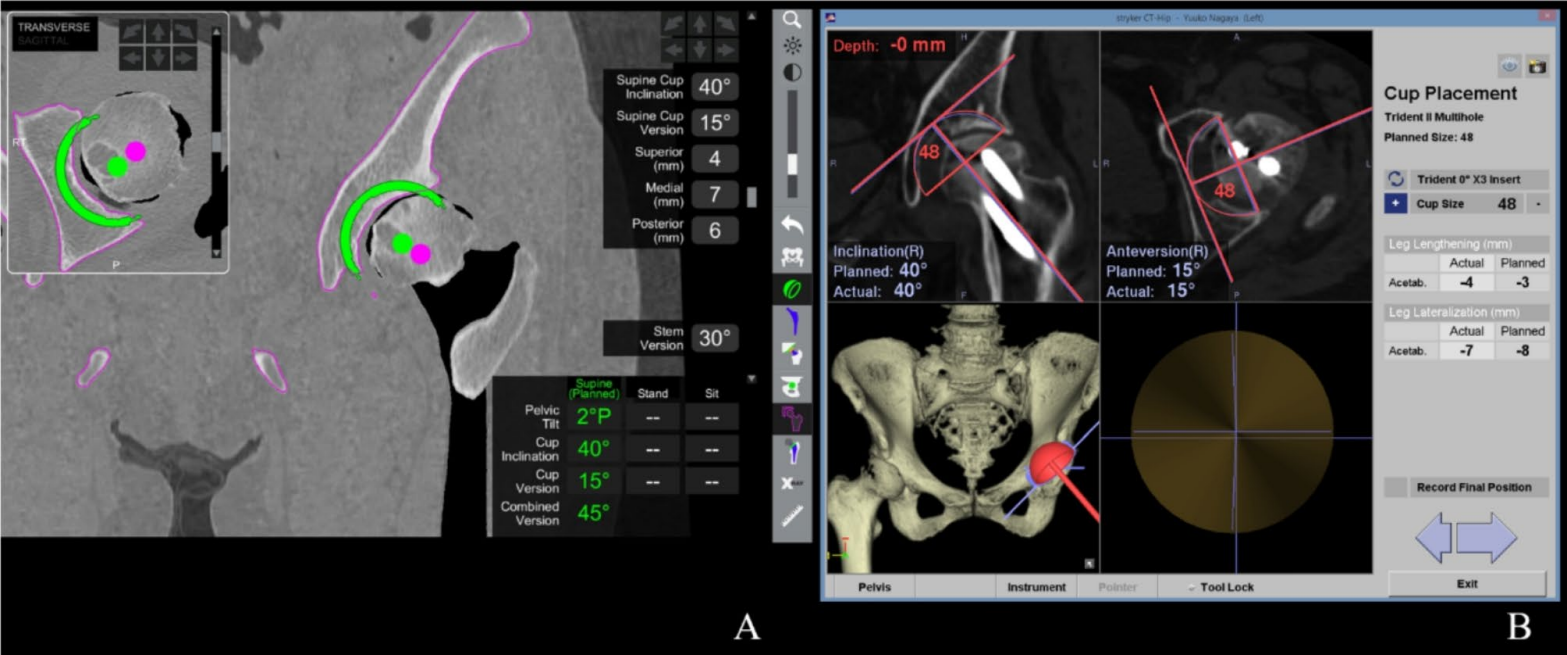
Acetabular component orientation and positioning (A) Robotic arm-assisted system and (B) CT-based navigation system
